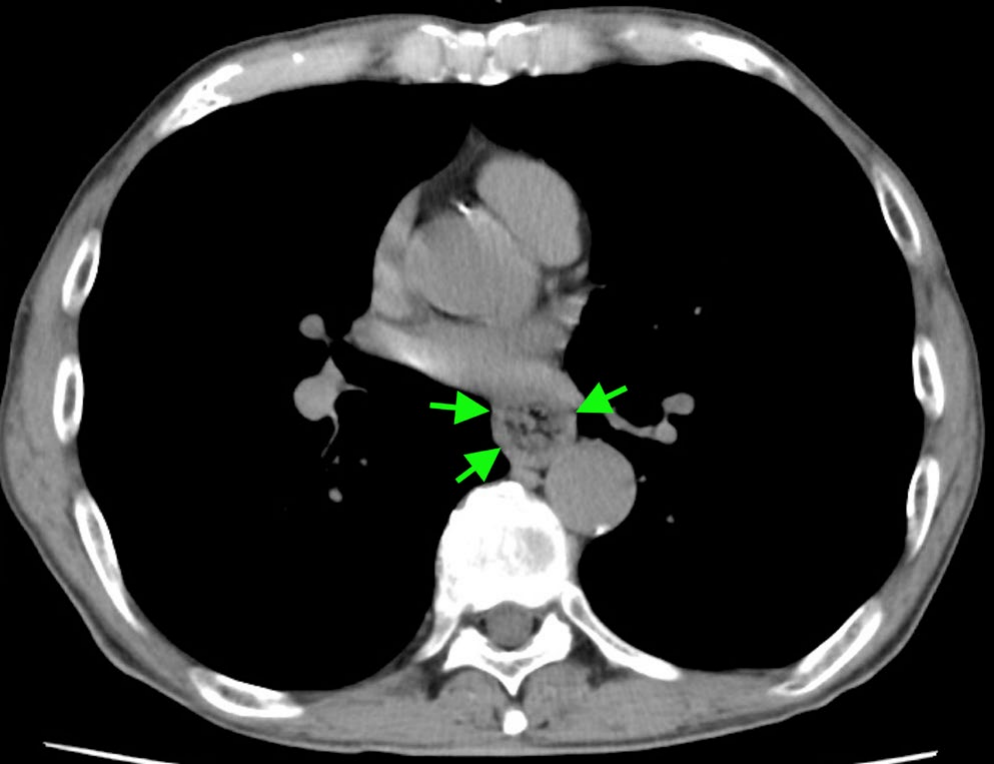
Computed tomography revealed circumferential esophageal wall thickening (arrows)
